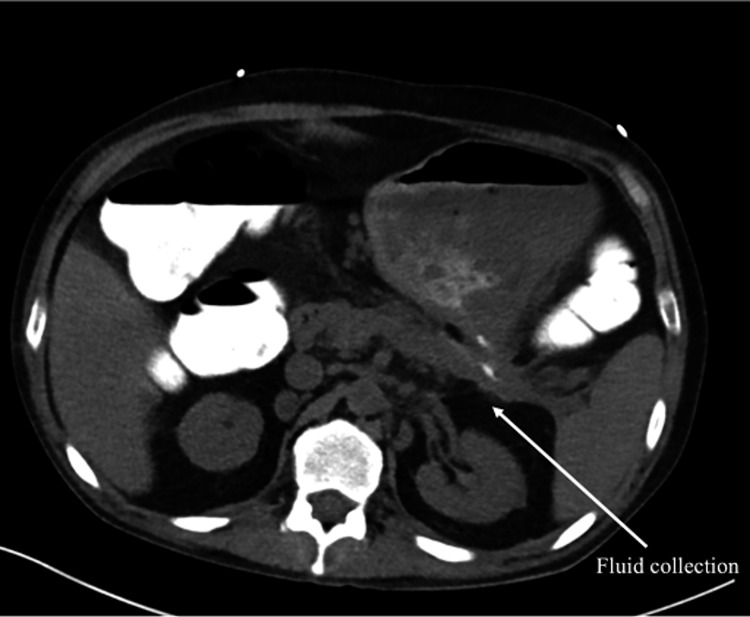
Axial CT scan with rectal contrast demonstrating extravasation of contrast (arrow) from the splenic flexure of the colon into a retroperitoneal inflammatory collection, confirming a pancreatico-colonic fistula
